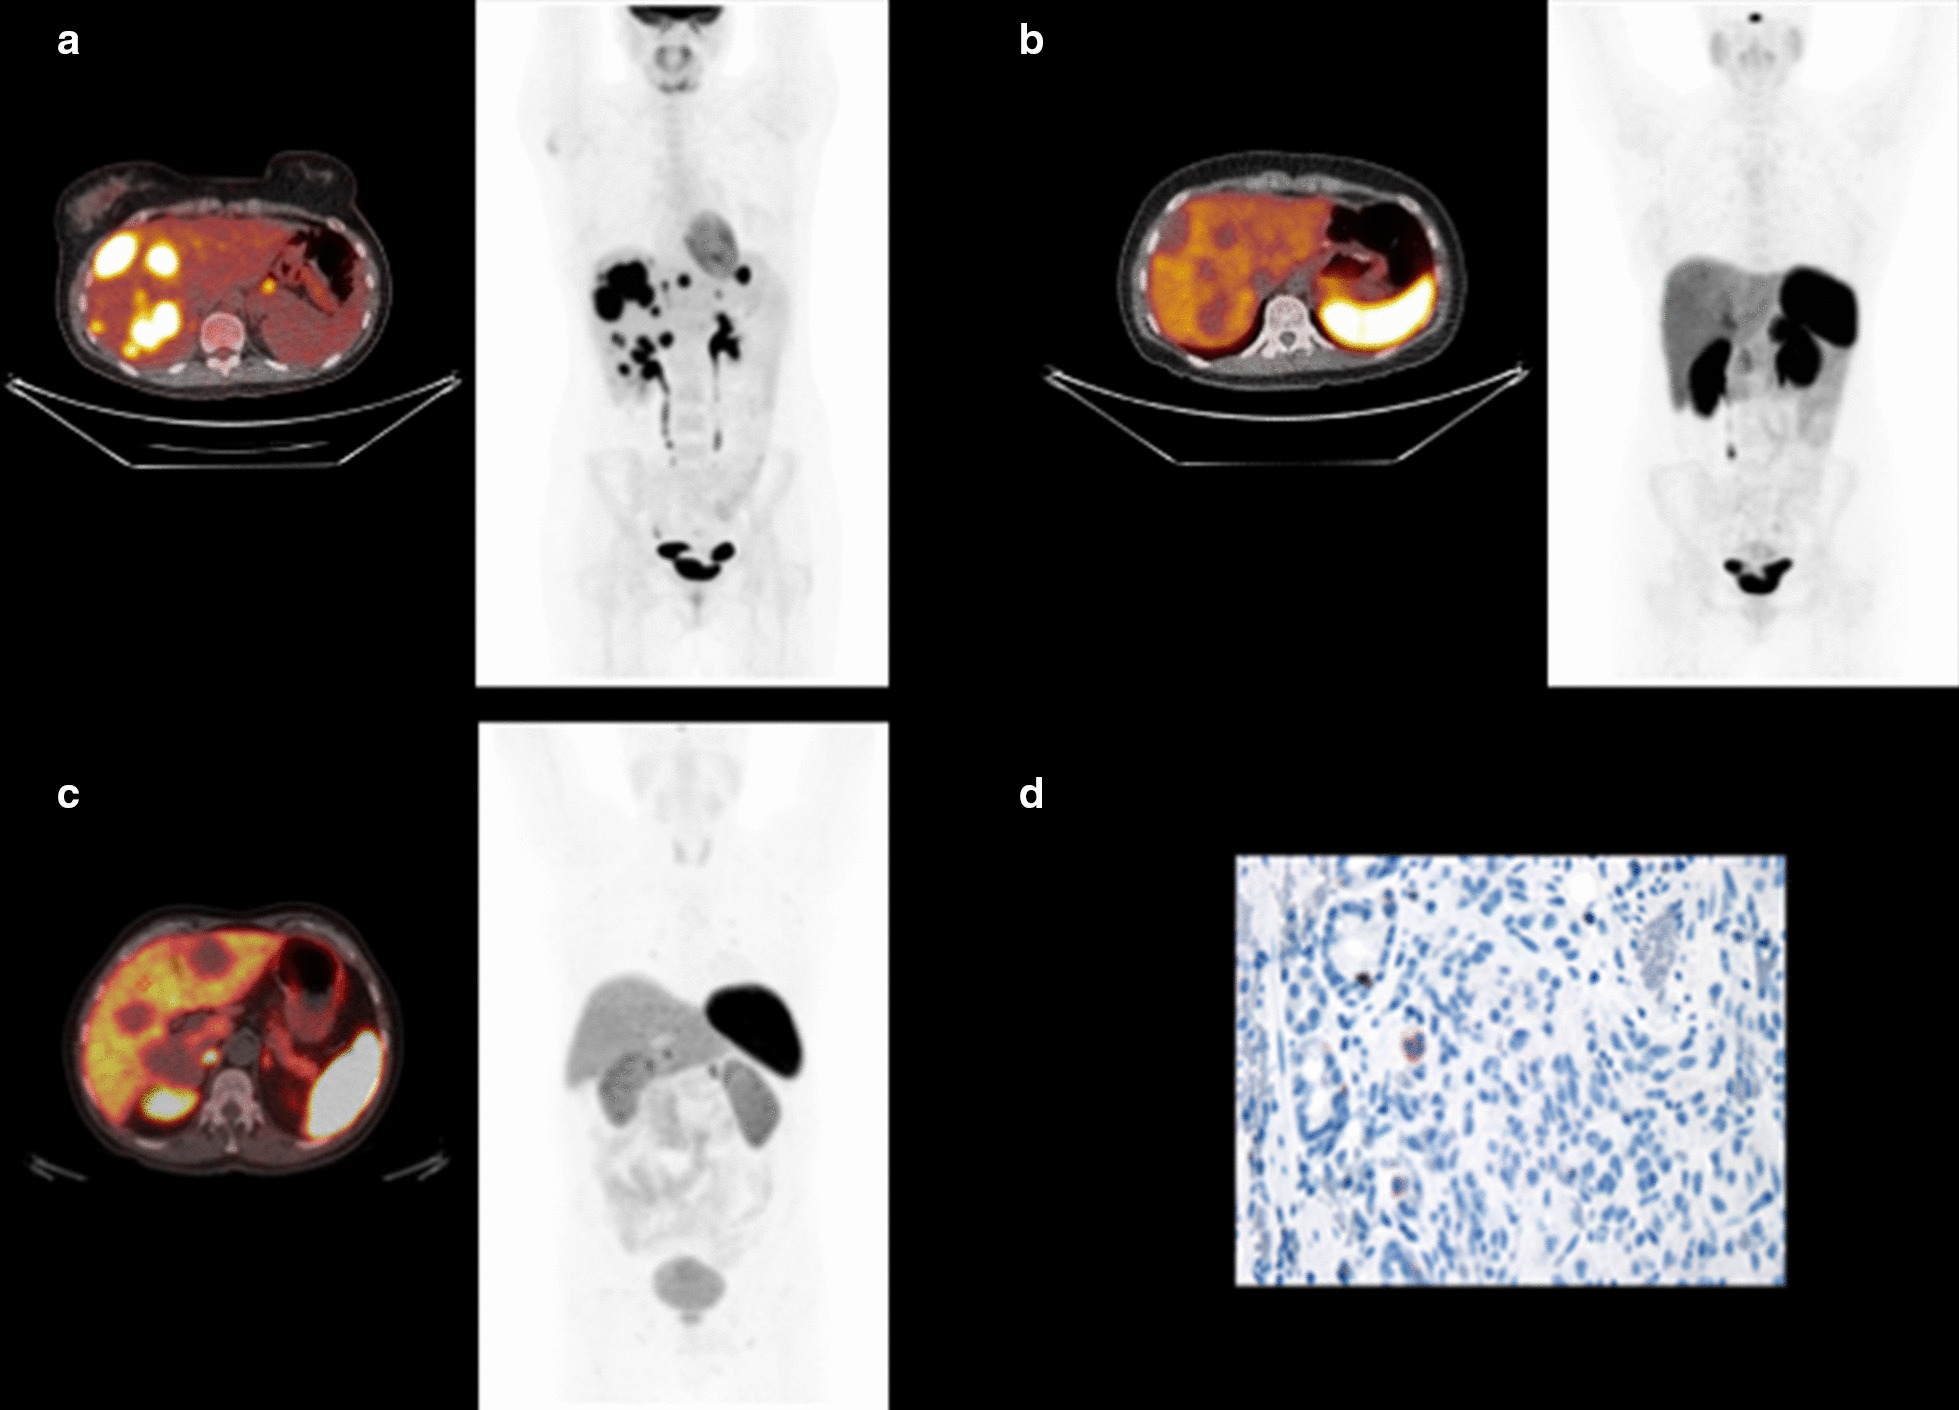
Panel A shows an axial fused [^18^F]FDG PET/CT image of the liver with a maximum intensity projection (MIP) image demonstrating highly FDG-avid liver and nodal metastases in case 001. Panel B shows the corresponding (case 001) trans-axial fused image of the [^68^ Ga]Ga-DOTATATE PET/CT and MIP image demonstrating low tracer uptake in the liver metastases (SUVmax 3) compared to background liver. Panel C, fused transaxial [^68^ Ga]Ga-DOTATATE PET/CT image and corresponding MIP image showing non-avid liver metastases in case 010 (SUVmax 2.5). Panel D shows negative SSTR2 expression of a liver metastasis on IHC for case 010
